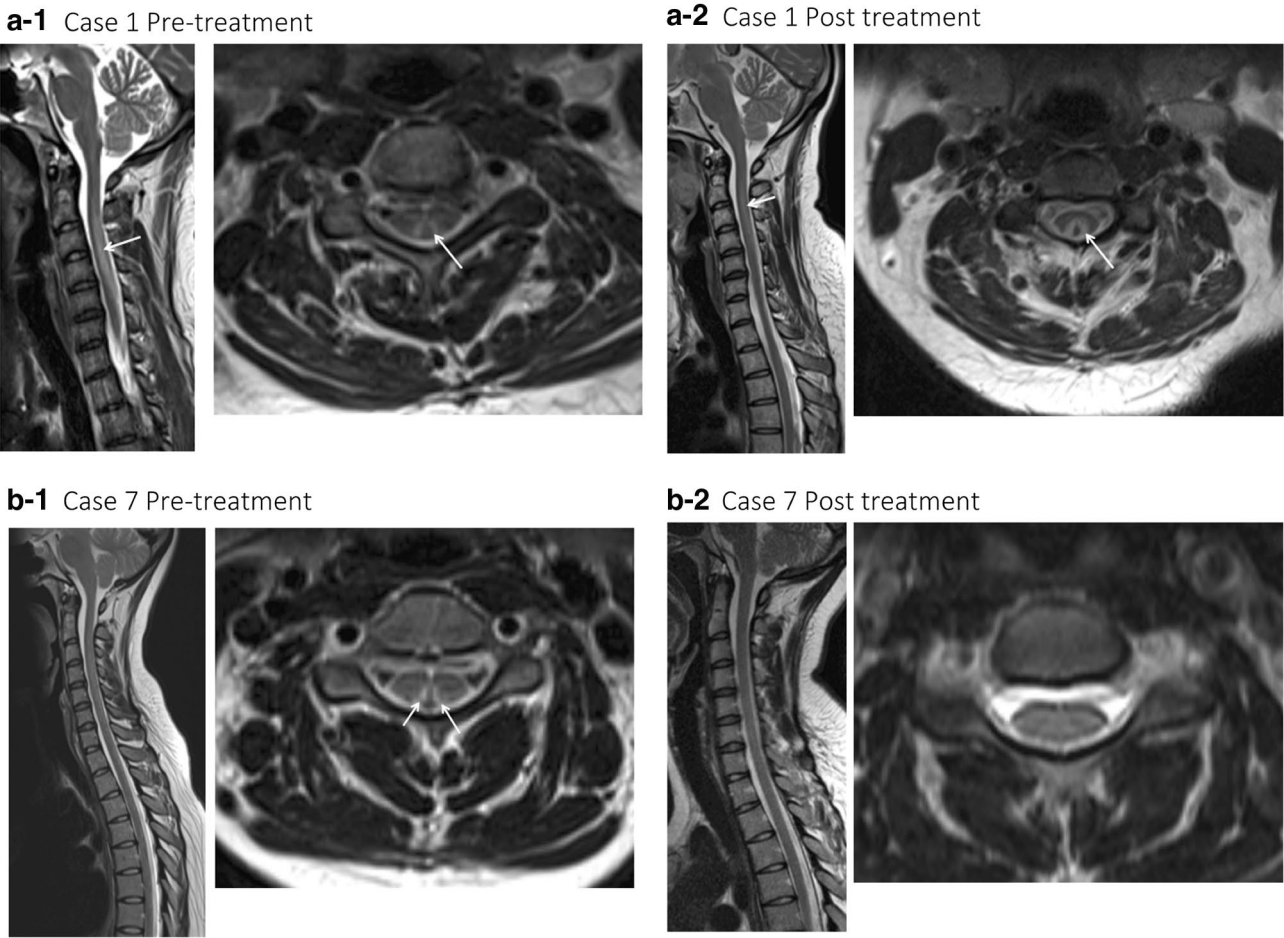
Pre- and post-treatment sagittal and axial T2 weighted MRI imaging of the cervical cord in N_2_O-induced subacute degeneration of the spinal cord. a-1: Sagittal T2 weighted sequence demonstrating extensive cord signal change from the C2 to the C7 level within the dorsal aspect of the cord confirmed on the axial sequences, with enhancement (not shown). a-2: Taken 5 months following showing persistent abnormal T2 signal change within the dorsal columns on this sagittal T2 weighted sequence; however, the abnormal enhancement had resolved (not shown). b-1: Sagittal T2 weighted sequence demonstrating cord signal change from C3 to C5 within the dorsal aspect of the cord confirmed on the axial sequences, without enhancement, and resolution over time (b-2)
